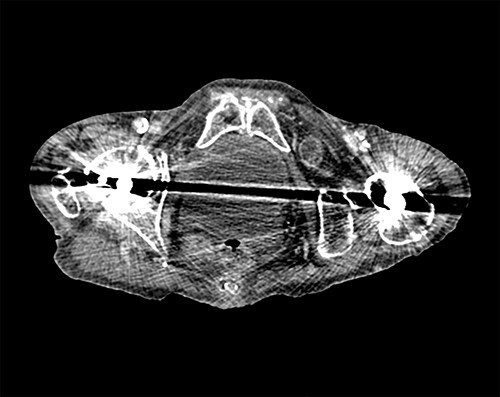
Axial view of CT scan showing the bowel loop through the left obturator hernia.
